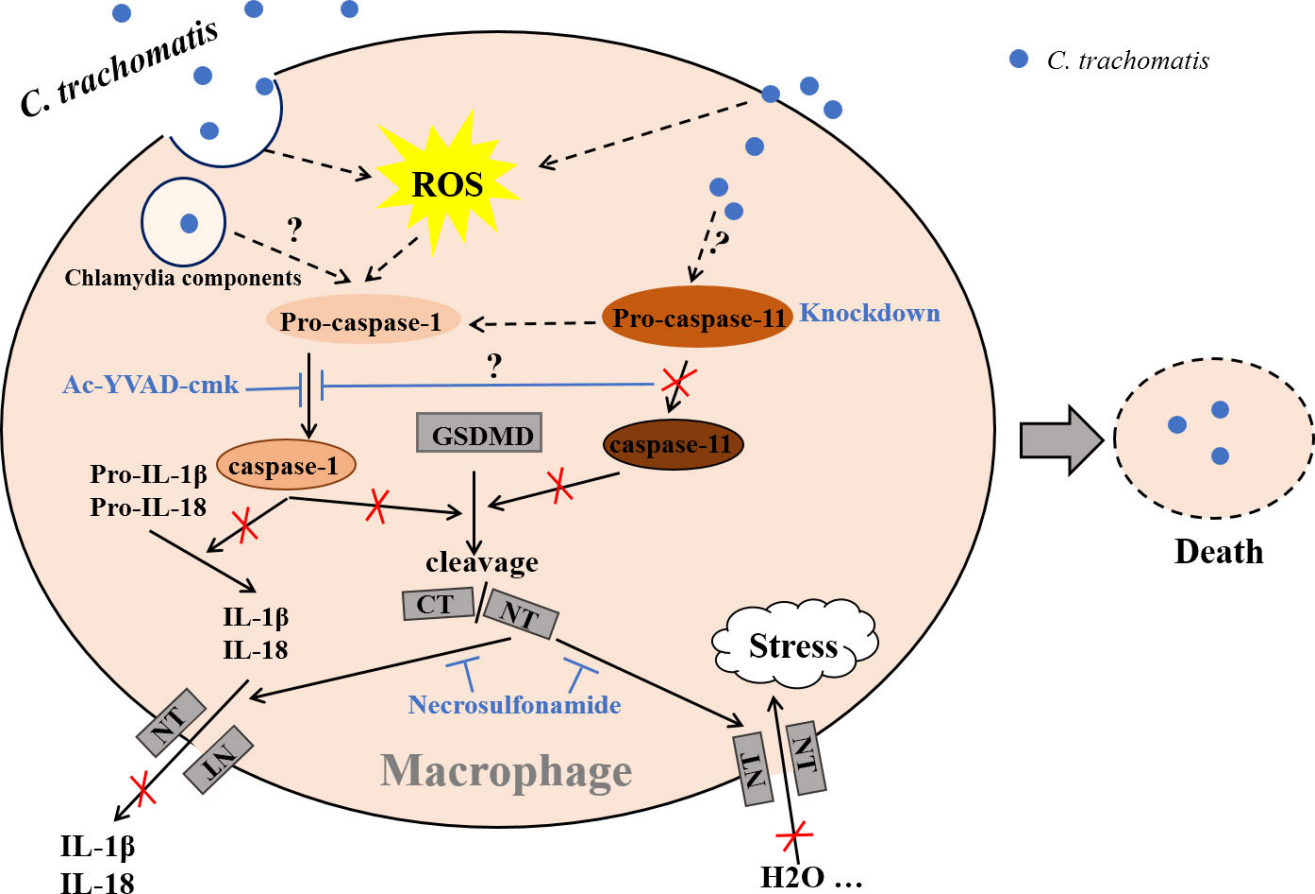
Proposed model of chlamydial growth restriction in macrophages by GSDMD-mediated pyroptosis. *C. trachomatis* is internalized by macrophages after contacting them during infecting host, cleaving GSDMD into GSDMD C-terminus (GSDMD-CT) and GSDMD-NT *via* activating caspase-1/11. GSDMD-NT could insert itself into the cell membrane to form pores. H2O influx leads to an increase in intracellular osmotic pressure, then causing cell swelling and rupture, destroying the replication niche of *C. trachomatis* to restrict its growth in macrophages. Active caspase-1 cleaves Pro-IL-1β and Pro-IL-18 into mature IL-1β and IL-18. Both are then released into the outside of cells during pyroptosis. Caspase-1 activation inhibition with Ac-YVAD-cmk blocks IL-1β and IL-18 mature and GSDMD cleavage. Caspase-11 knockdown inhibits both GSDMD activation and caspase-1 activation. Necrosulfonamide restrains treatment GSDMD-NT oligomerization and pyroptotic pore formation, inhibiting IL-1β and IL-18 secretion and H2O influx. Dashed lines represent published pathways, solid lines represent pathways described in this study. It is currently unknown what *C. trachomatis* components activate caspase-1 or caspase-11(not by Chlamydia LPS). It is unclear how caspase-11 signal pathway interacts with caspase-1 signal pathway.
